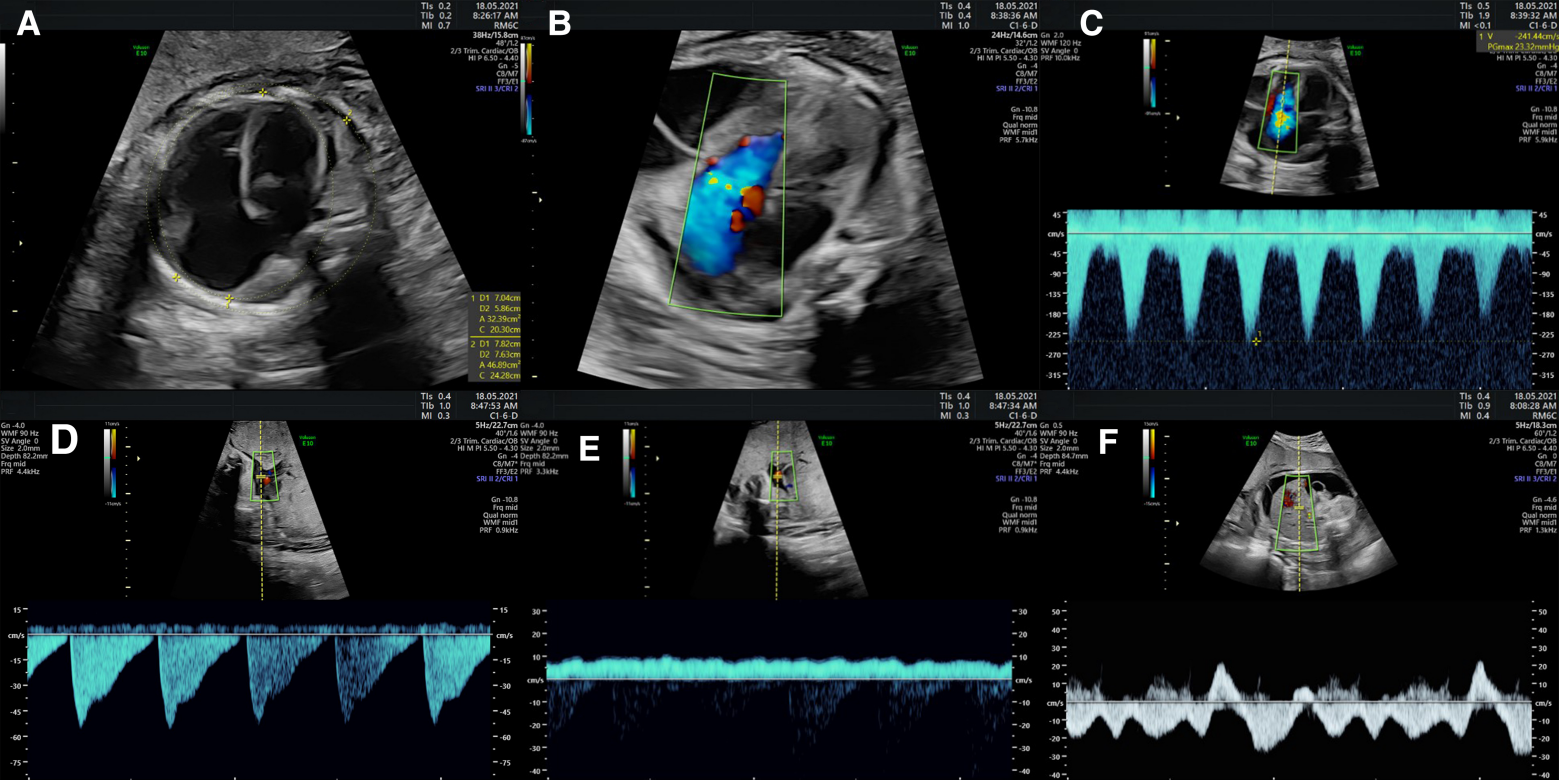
The assessment of fetal cardiac function and cardiovascular profile in Ebstein anomaly showing severe cardiomegaly (cardiothoracic ratio: 0.69) with severely dilated right atrium and right ventricle and compressed lungs and mild to moderately reduced RV function, fetal hydrops with a small pericardial effusion, significant ascites and scalp edema. The Dopplers reveal intermittent a-wave reversal in the ductus venosus and umbilical vein notching suggestive of elevated cardiac filling pressures and intermittently absent diastolic flow in the umbilical artery consistent with a systemic steal. (A) Four chamber view suggesting severe cardiomegaly (cardiothoracic ratio: 0.69) and dilated right heart. (B) Color Doppler showing tricuspid regurgitation. (C) Doppler of tricuspid valve showing biphasic flow with high velocity. (D) Intermittently absent diastolic flow in the umbilical artery. (E) Umbilical vein notching. (F) The intermittent a-wave reversal in the DV.
